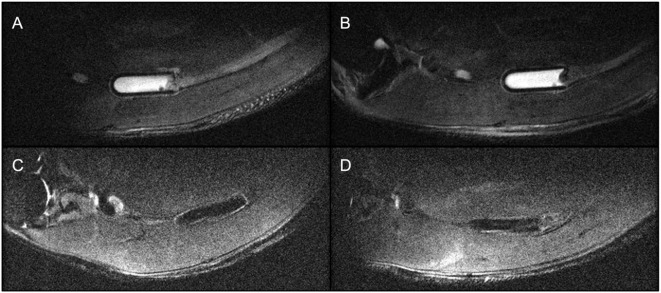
MRI evaluation of cap degradation. T2-weighted MRI images showed that chitosan caps displayed hardly any degradation from 6 weeks (A) and 12 weeks (B) after surgery. The polylactide caps showed little degradation from 6 weeks (C) to 12 weeks (D) after surgery.
